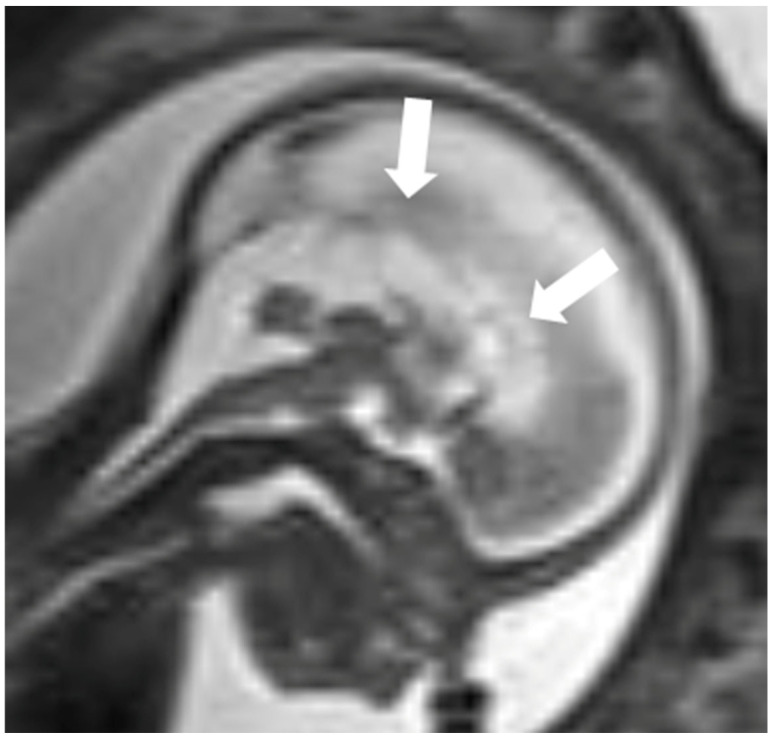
Fetal MRI sagittal T2W sequence at 21st week of gestation shows agenesis of corpus callosum (white arrow).
